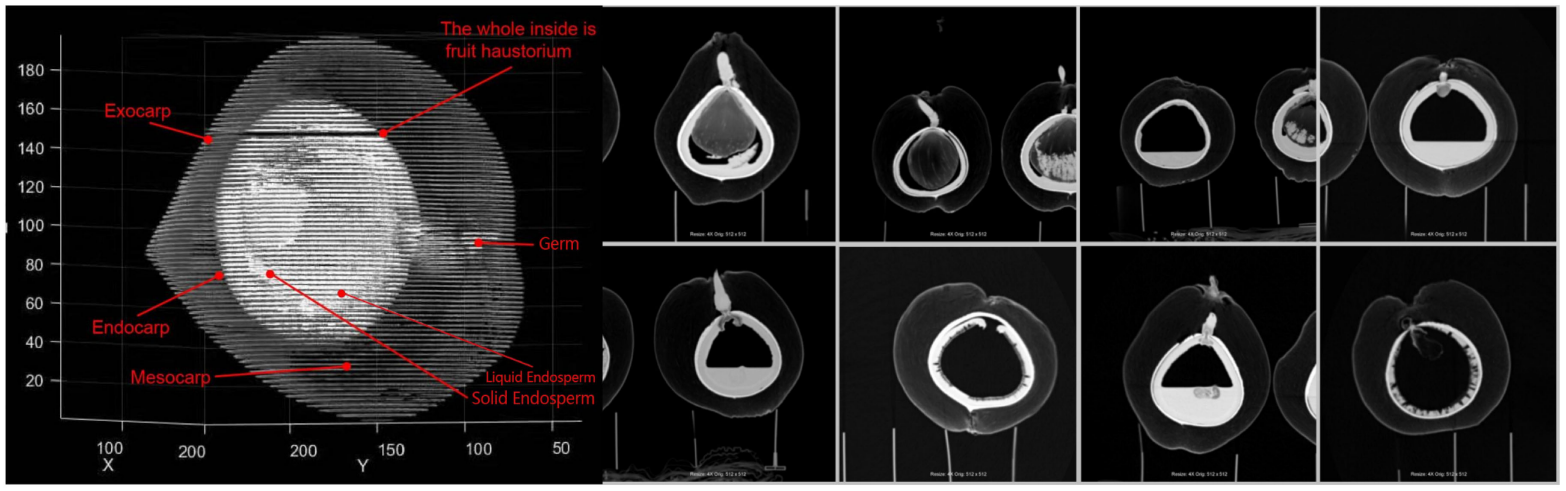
Example of CT image of coconut.
